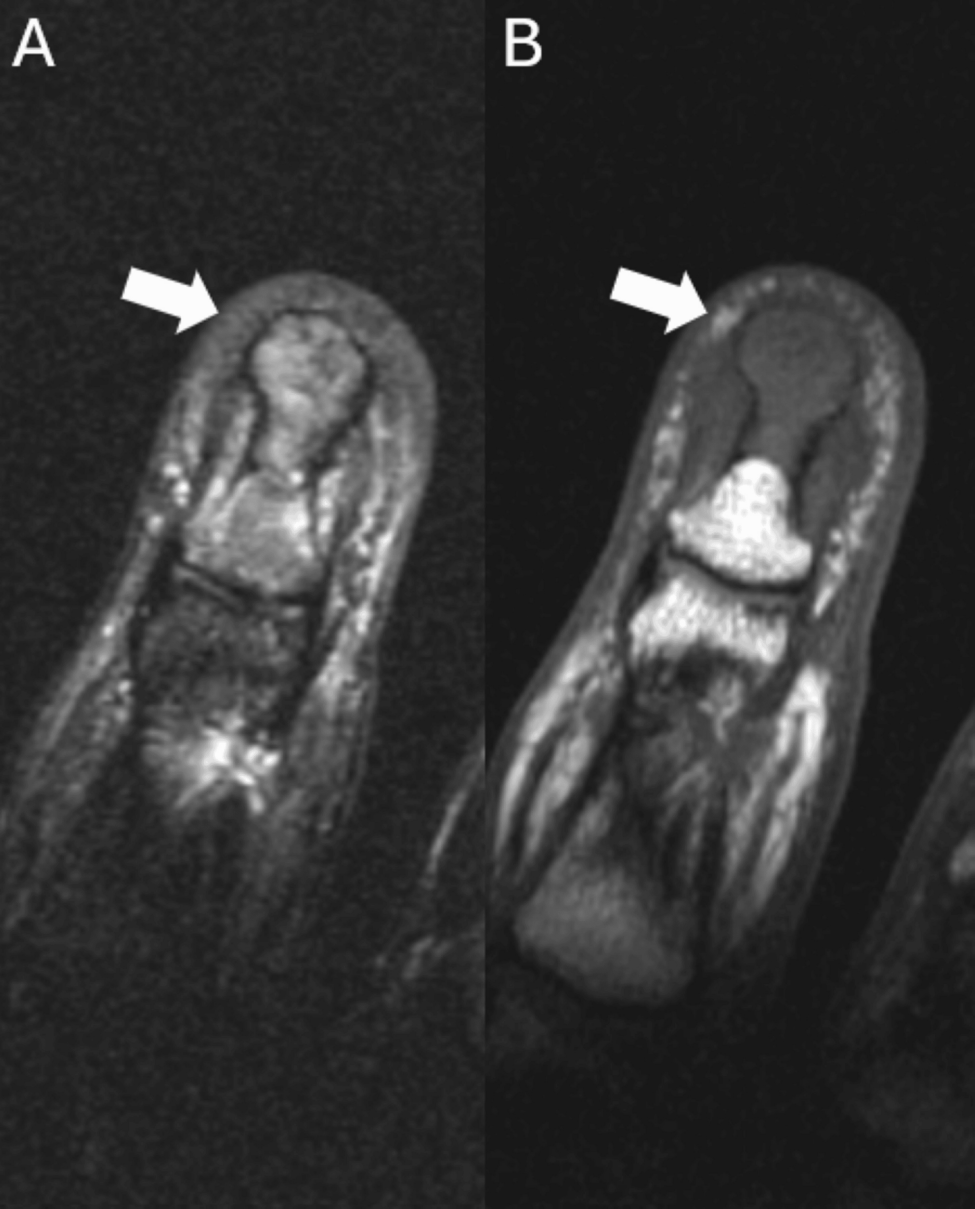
A) Coronal STIR MRI of the left third distal phalanx, demonstrating an intraosseous mass with an expansile bulbous appearance at the tuft. B) Coronal T1 MRI of the left third distal phalanx, revealing no edema within the mass and the adjacent normal bone. STIR: Short tau inversion recovery
